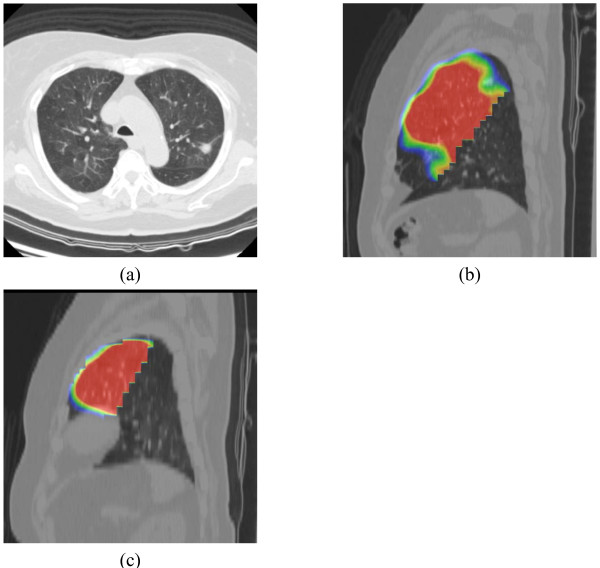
Images of before and after segmentectomy. (a) Axial image of CT before surgery, showing lung cancer in posterior apical segment of the left upper lobe. (b) Sagittal image of the lung-perfusion single-photon-emission computed tomography and CT (SPECT/CT) of the left upper lobe before operation. (c) Sagittal image of the lung-perfusion SPECT/CT of the remaining lingular segment after resection of upper division segmentectomy.
